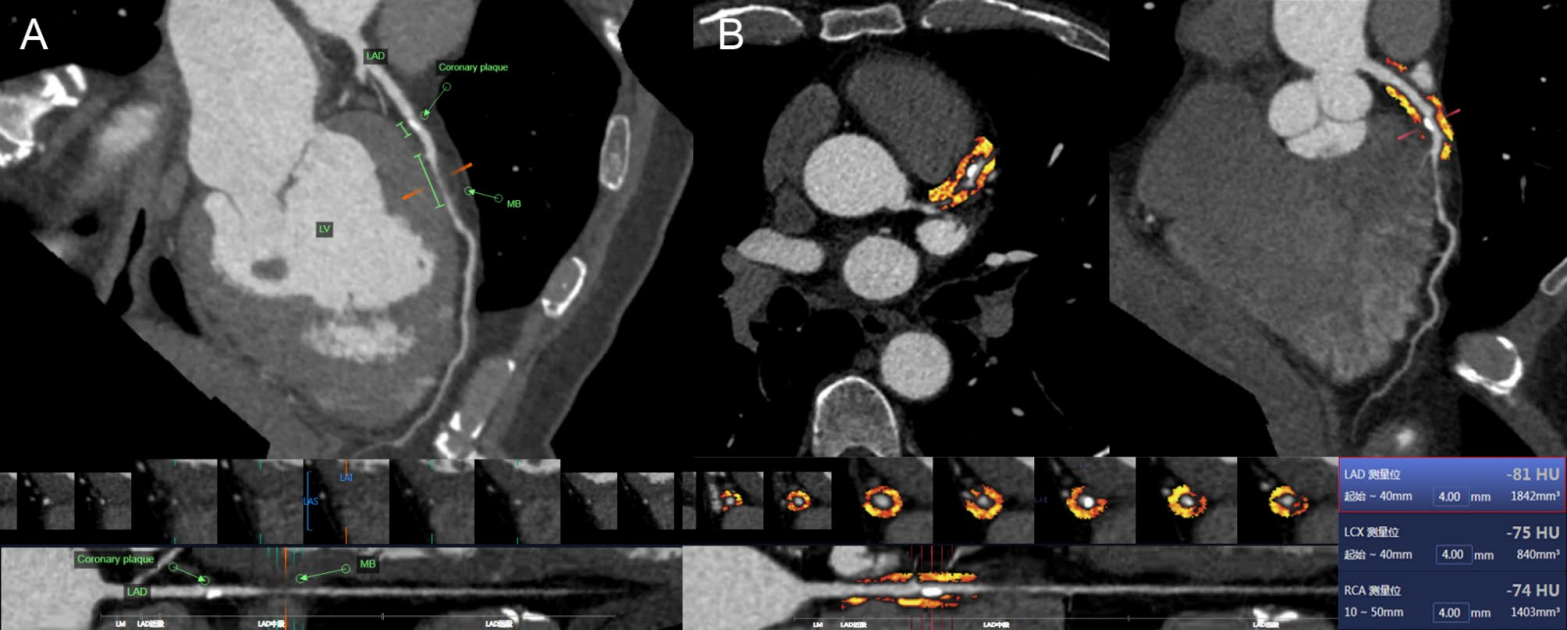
A 68-year-old male underwent CCTA. (a) The patient had an MB on the left anterior descending artery, and coronary artery stenosis covered by the MB was obvious. The patient had coronary plaque in the segment proximal to the MB. (b) The FAI_lesion_ value for the 40-mm segment around the coronary plaque proximal to the MB was − 81 HU (the red frame). *CCTA*: coronary computed tomography angiography, *MB*: myocardial bridge, *FAI*: fat attenuation index
